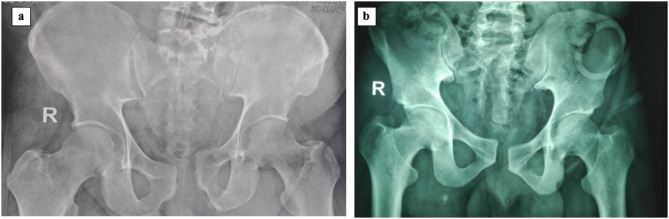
Shows preoperative x-rays that show a-Tile C1-2 and b- C1-3 injuries, respectively
